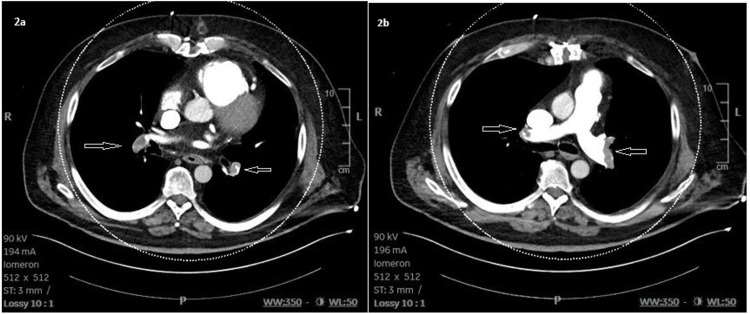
CTPA with central emboli Bilateral pulmonary embolism with intraluminal filling defects (yellow arrows). 2a: Thrombus is visible in the right and left interlobar pulmonary arteries. 2b: Thrombus is visible in both the right and left pulmonary arteries, consistent with a proximal pulmonary embolism. CTPA: computed tomography pulmonary angiography
